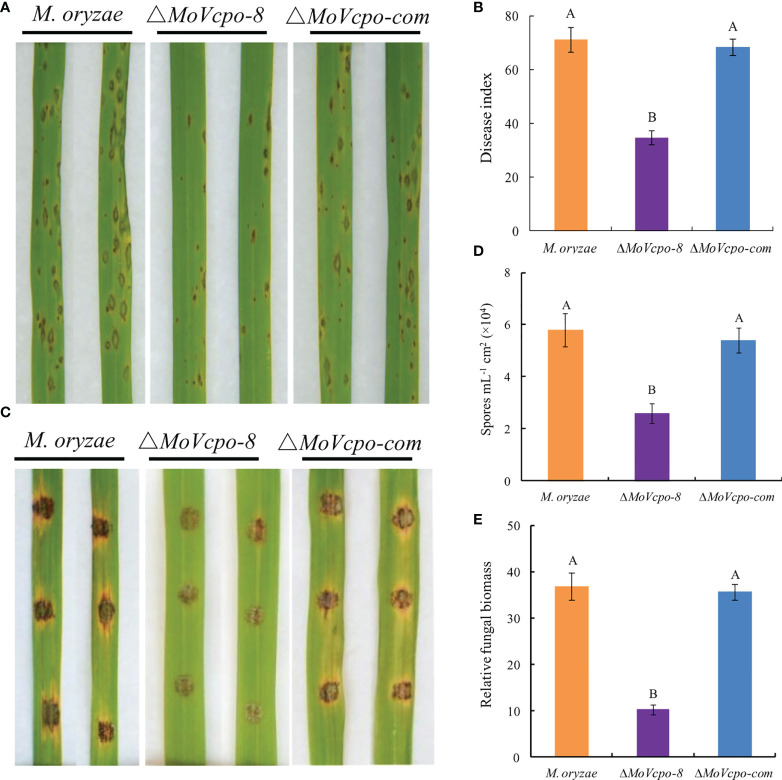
Pathogenicity assay of *MoVcpo* deletion mutant and complementation strain. (A) Virulence bioassay on rice cultivar cv. CO39 conducted by the spraying inoculation method. The symptoms of rice plants were investigated 7 dpi and photographed. (B) Disease index of rice blast in spraying inoculation bioassay. (C) Virulence bioassay on rice plants conducted by the punch inoculation method. Leaves were photographed 10 dpi. (D) Sporulation on the punch-inoculated leaves. Samples were taken for the assays 10 dpi. (E) Relative biomass assay on the punch-inoculated leaves. The relative fungal growth was measured by [2^[CT(^
